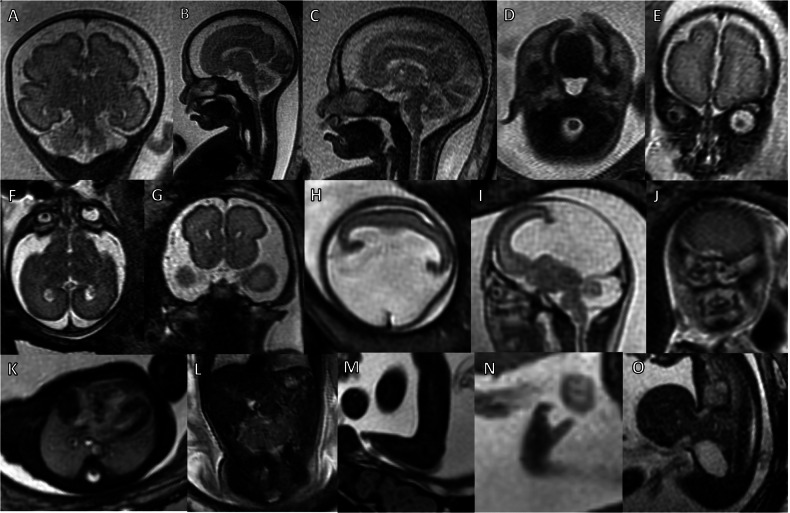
MR phenotyping CHARGE syndrome and trisomy 13 and 18. First row (A–E): CHARGE syndrome A, widened external CSF spaces (29 + 1 weeks gestational age [GW], T2-weighted MRI); B esophageal atresia with cervical pouch sign (29 + 1 GW, T2); C + D cleft lip and palate (29 + 1 GW, T2); E aplastic olfactory bulbi (29 + 5 GW, T2). Second row (F–J): trisomy 13 F, microphthalmia (28 + 3 GW, T2); G, microtia (28 + 3, T2); H–J, alobar holoprosencephaly with microphthalmia and choanal atresia (21 + 5 GW, T2). Third row (K–P): trisomy 18. K ventricular septum defect (32 + 0 GW, steady-state free precessing MRI-SSFP); L horseshoe kidney (32 + 0 GW, SSFP); M rocker-bottom feet (32 + 1 GW, SSFP); N ectrodactyly (23 + 3 GW, T2); O omphalocele (29 + 1 GW, SSFP)
